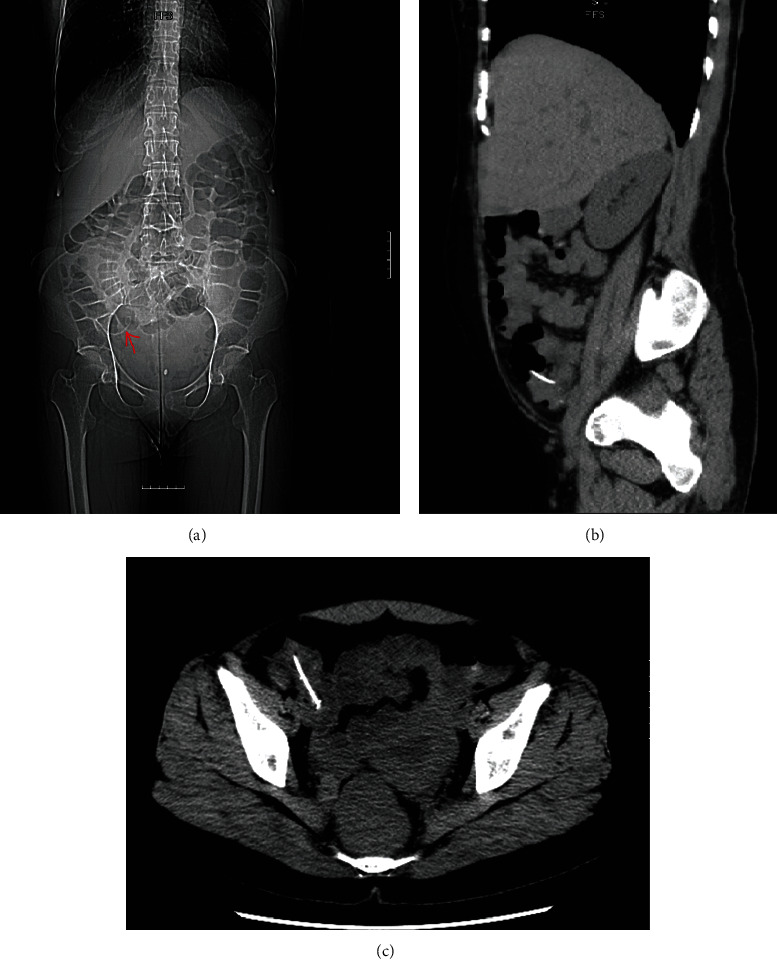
The CT scan showing a portion of the pigtail catheter lodged within the cecum in a coronal view (a), sagittal view (b), and axial view (c).
